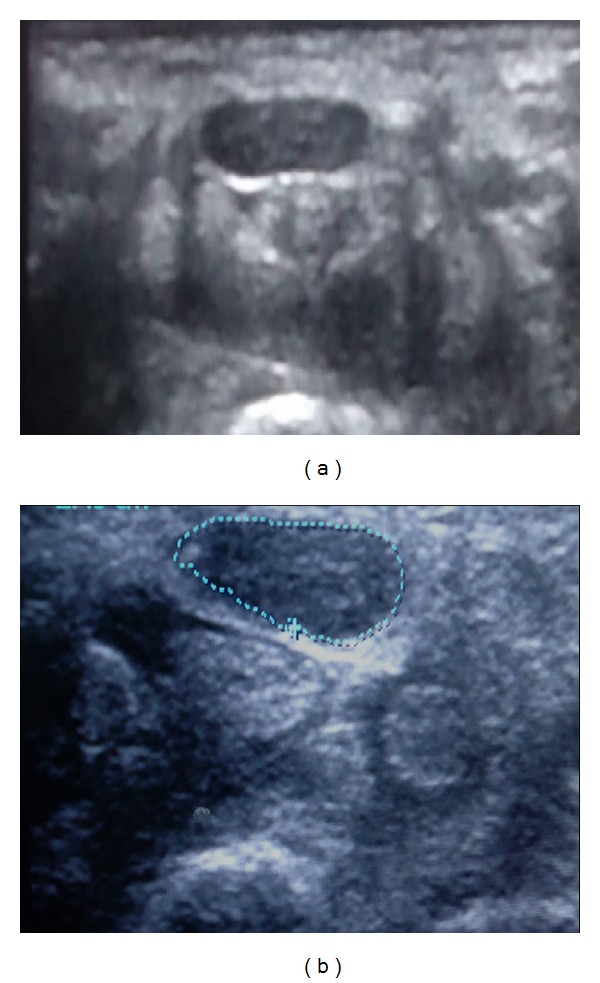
(a) Wrist ultrasound at the carpal tunnel inlet for patient with severe CTS medial, (b) the tracing method for measuring the cross sectional area of the median nerve which was 18 mm^2^.
